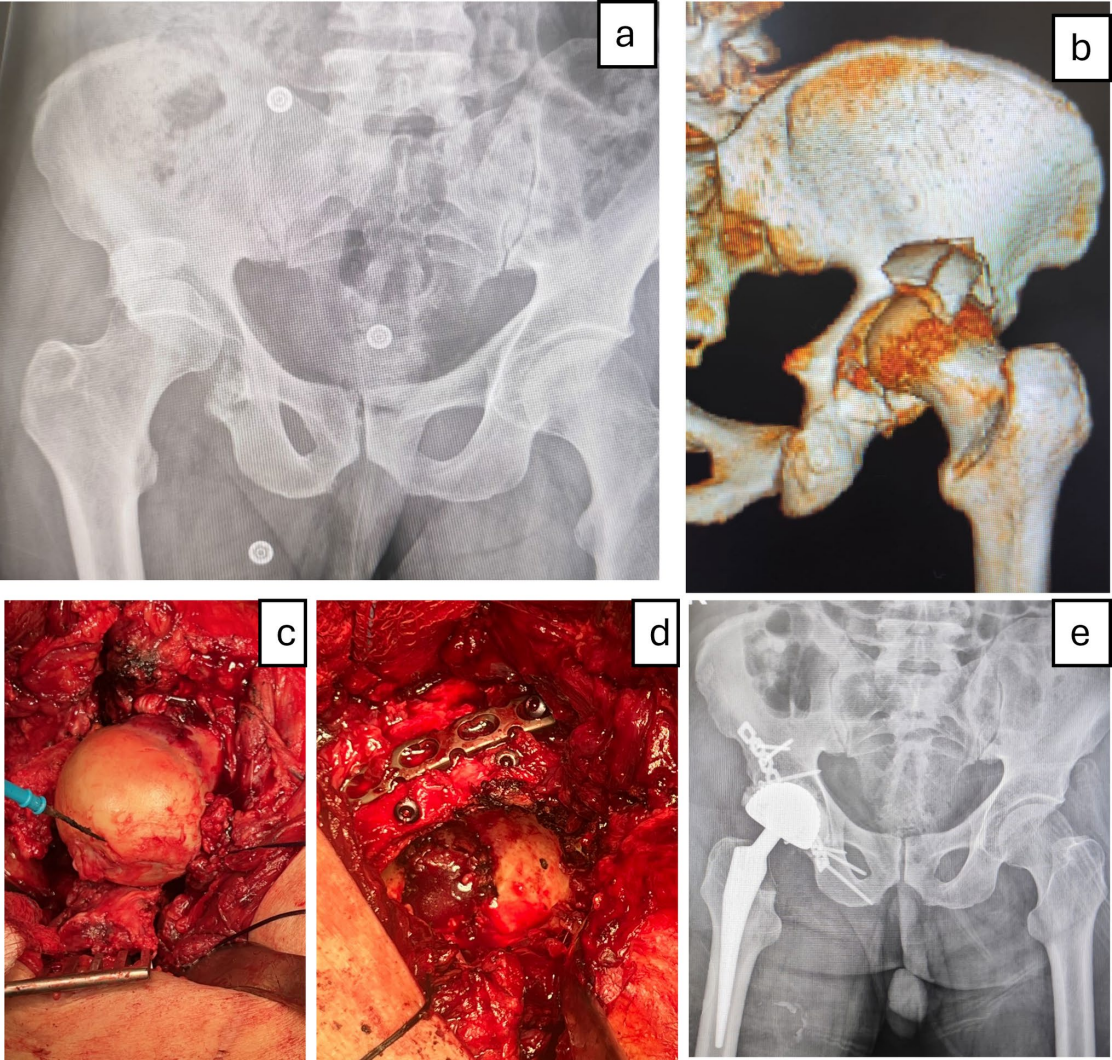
a, b Preoperative radiograph and 3D CT scan demonstrating a 4-week neglected comminuted posterior wall fracture with hip dislocation. c, d Intraoperative photographs showing an impaction fracture of the femoral head and fixation of the comminuted posterior wall using lag screws and a reconstruction plate. e Final follow-up radiograph demonstrating a stable prosthesis with no evidence of loosening or radiolucent lines
